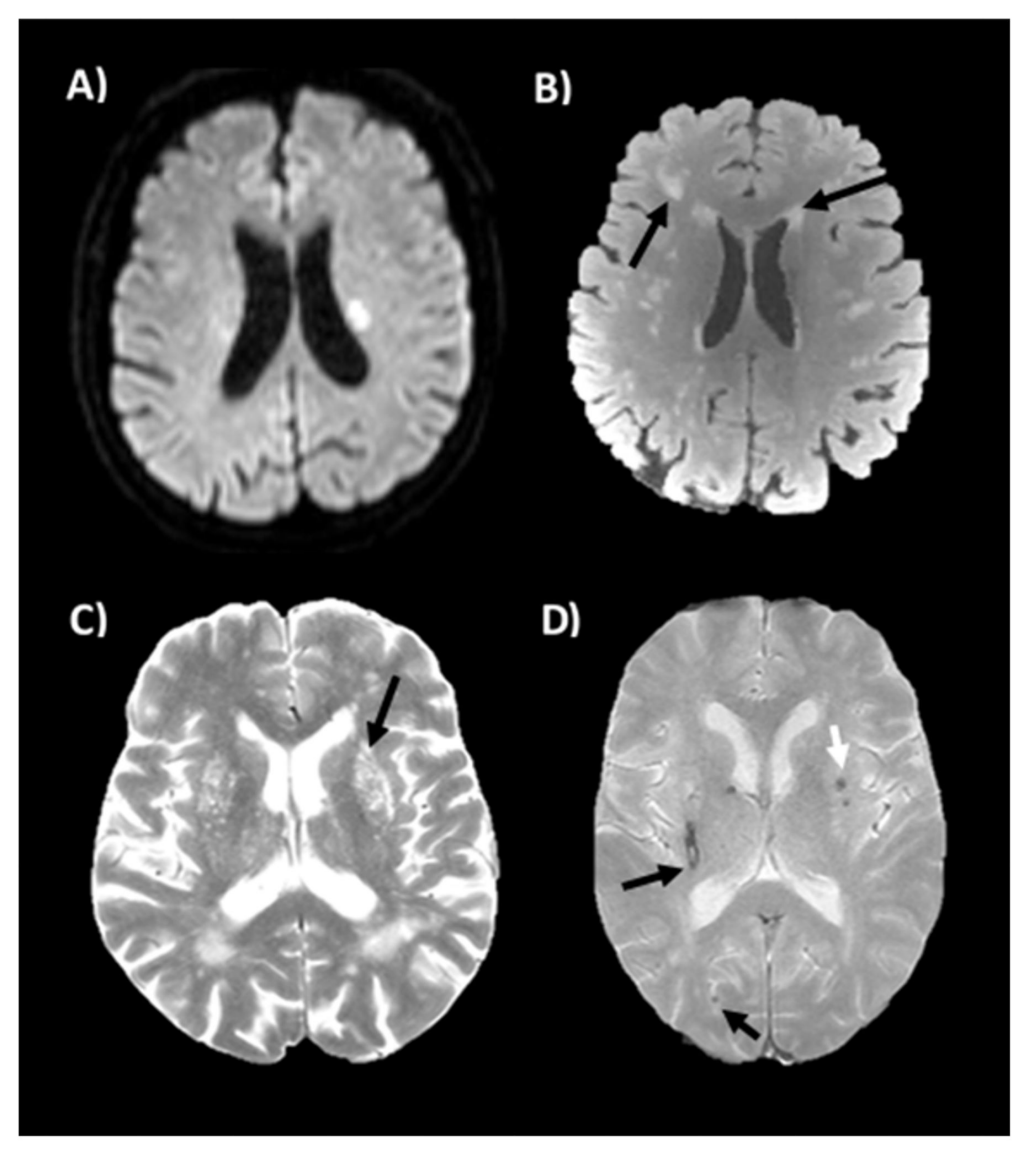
Radiological manifestations of small vessel disease. A) An acute small subcortical infarct on DWI imaging; B) Deep and periventricular white matter hyperintensities (arrowed); C) Dilated perivascular spaces bilaterally in the basal ganglia (arrowed on the left); D) a slit-like haemosiderin-lined cavity due to a chronic right intracerebral haemorrhage, subcortical (deep) cerebral microbleeds (white arrow) and a cortical (lobar) (black arrow) microbleed.
